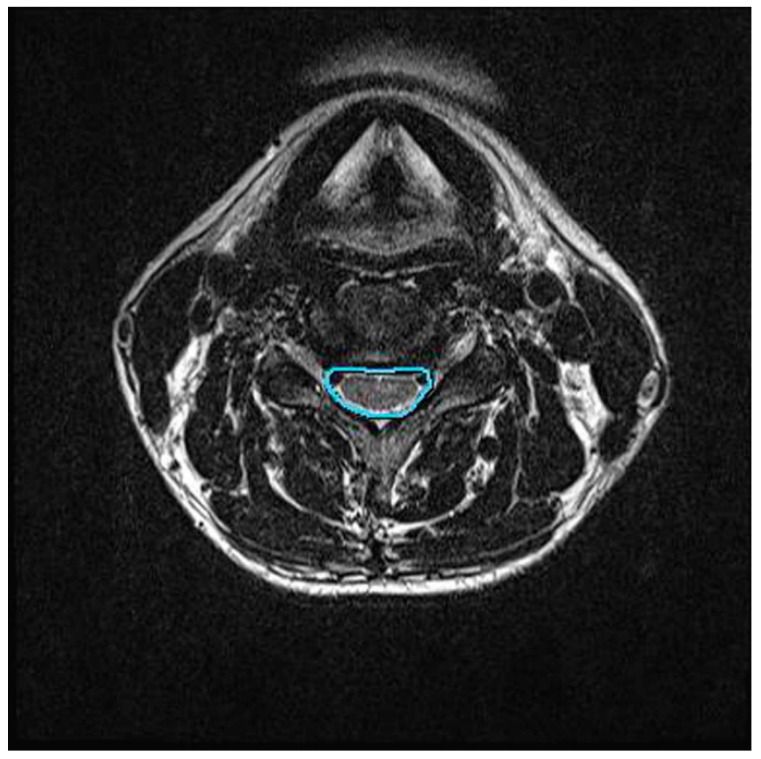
Central canal area in axial view of cervical spine MRI.
